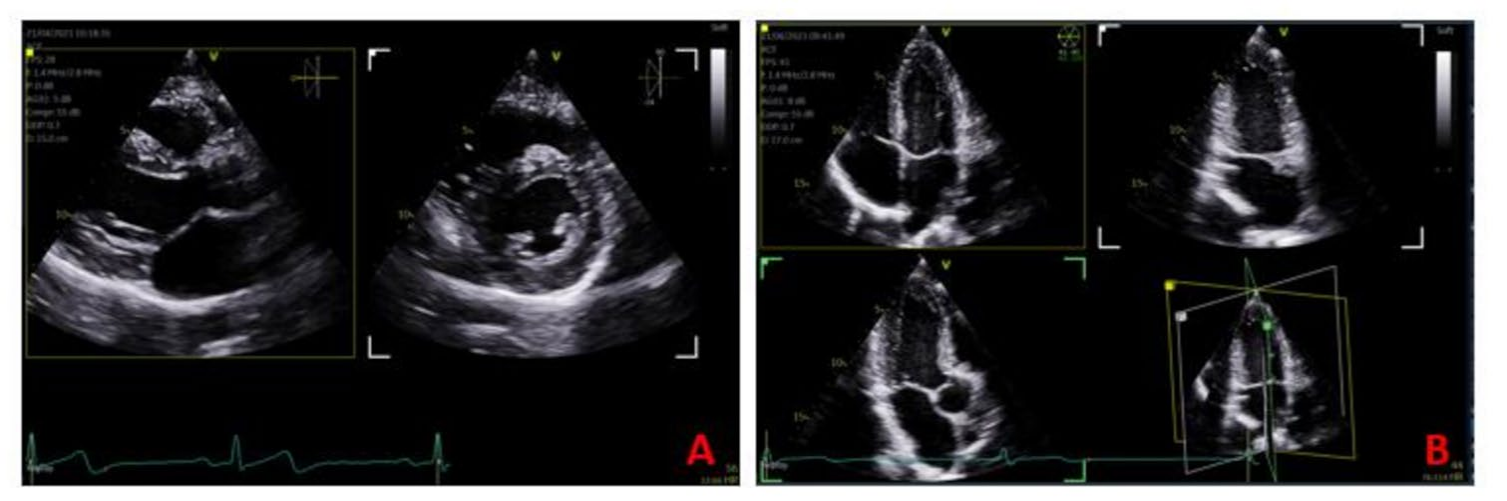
Biplane and Triplane imaging. A biplane image (A) of the parasternal view of the heart. The biplane cursor has been positioned at the level of the papillary muscles in the long axis view to display the corresponding short axis image on the right. The triplane image (B) displays the original scan plane of the apical 4 chamber view on the top left. The triplane angle has been adjusted to display the on-axis apical two chamber view (top right-hand panel) and apical long axis view (bottom left-hand panel)
